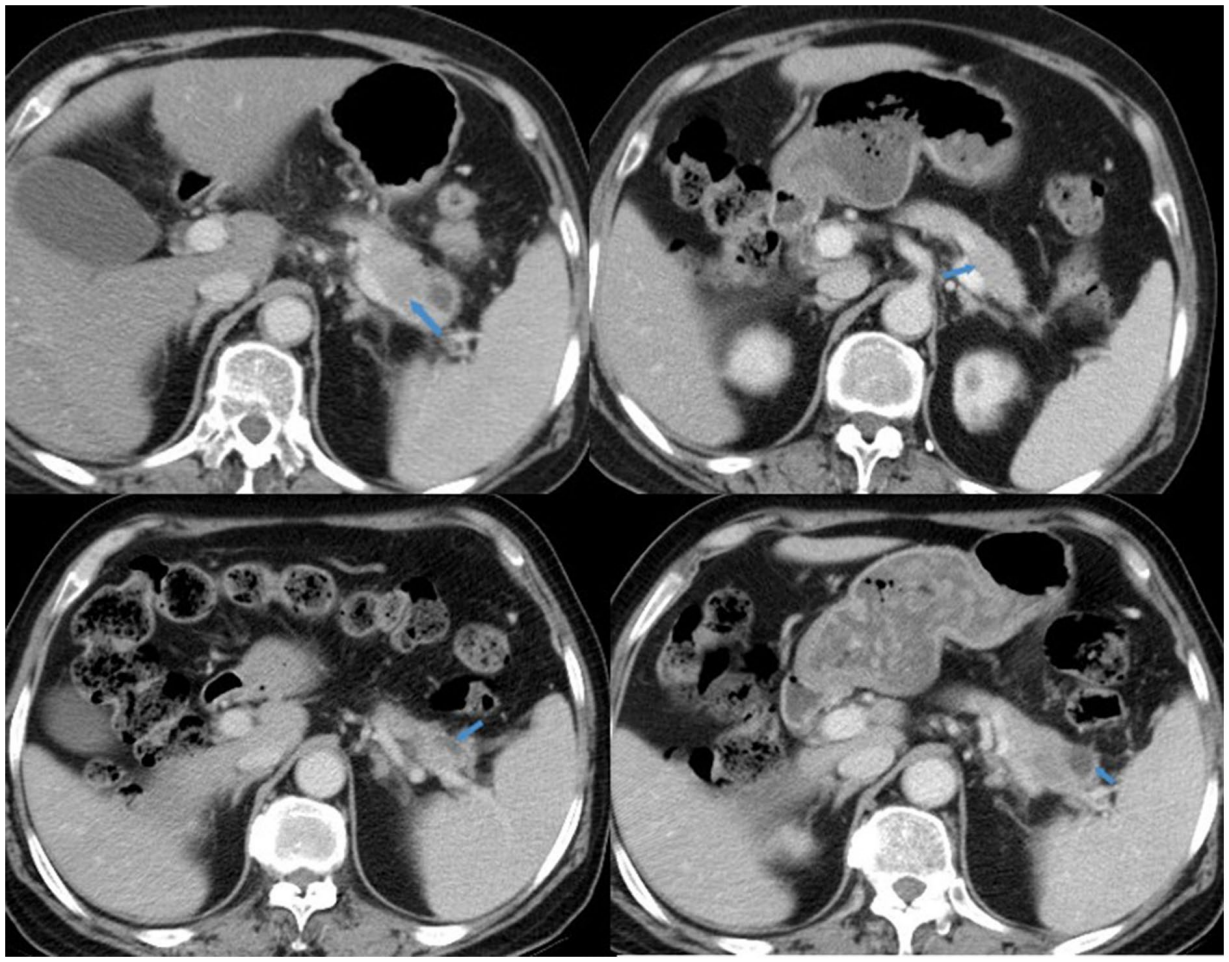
A 65-year-old woman with lung cancer. She received a docetaxel and cisplatin regimen for two cycles, with AMY = 449 U/L. CT imaging showed pancreatic changes, from heterogeneous enhancement (2019-5-31, upper right) to lesion area reduction and improvement (2019-12-31, upper left), followed by eventual pseudocyst formation (2020-3-19 and 2020-6-30, lower right and left).
